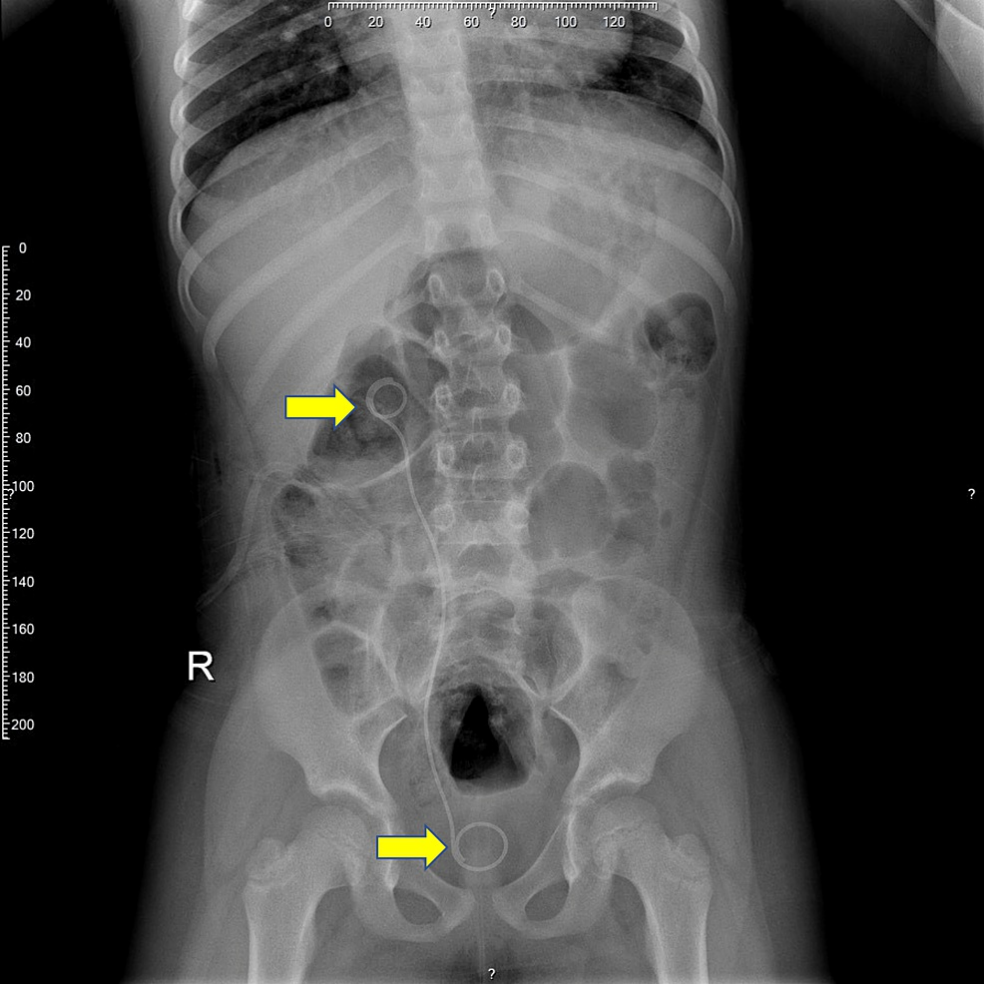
Postoperative x-ray showing the inserted double J stent (yellow arrows).
